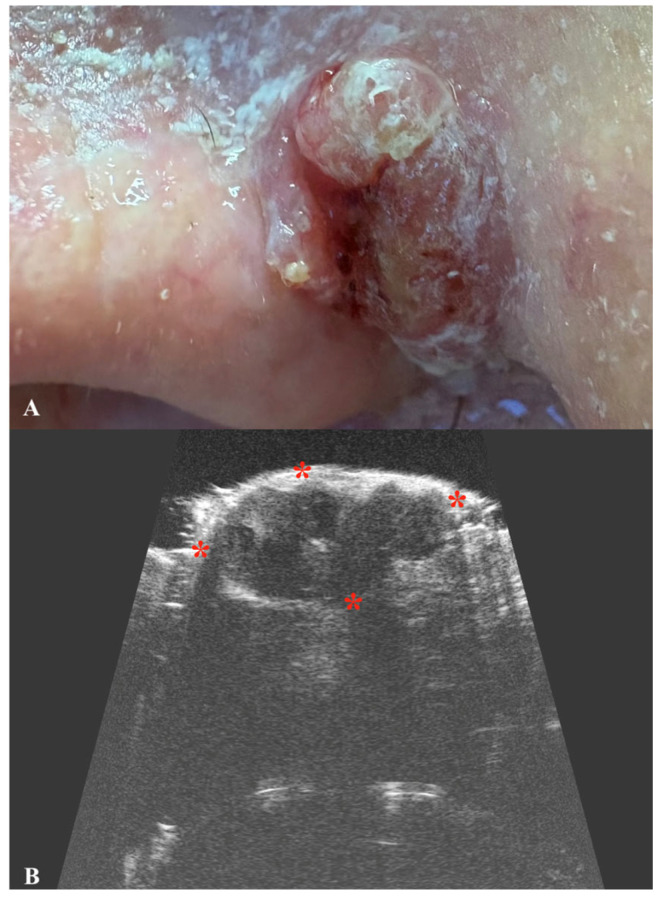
Macroscopic view of basal cell carcinoma (A) with a corresponding ultrasound image (B), presenting a hypoechoic nodule with a hyperechoic border and multiple intranodular hyperechoic dots. Stars indicate tumor margins.
